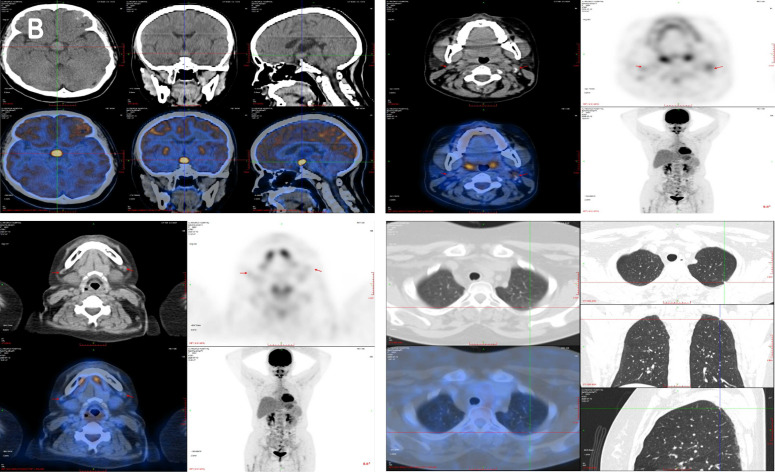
Postoperative whole-body PET–CT for systemic staging showing hypermetabolic lesions in the hypothalamic region, cervical and axillary lymph nodes, consistent with multisystem LCH.
